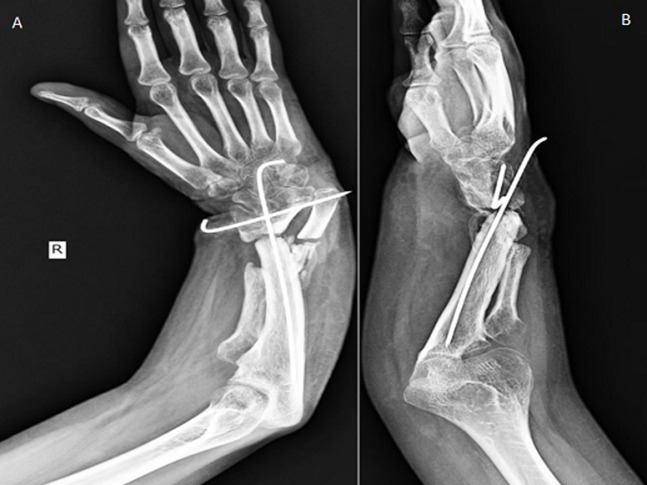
post-operative (osteotomy of radius and ulna) X-ray antero-posterior (A) and lateral (B) view of right forearm showing reduction of the deformity using a fibular bone graft and k-wire stabilization
